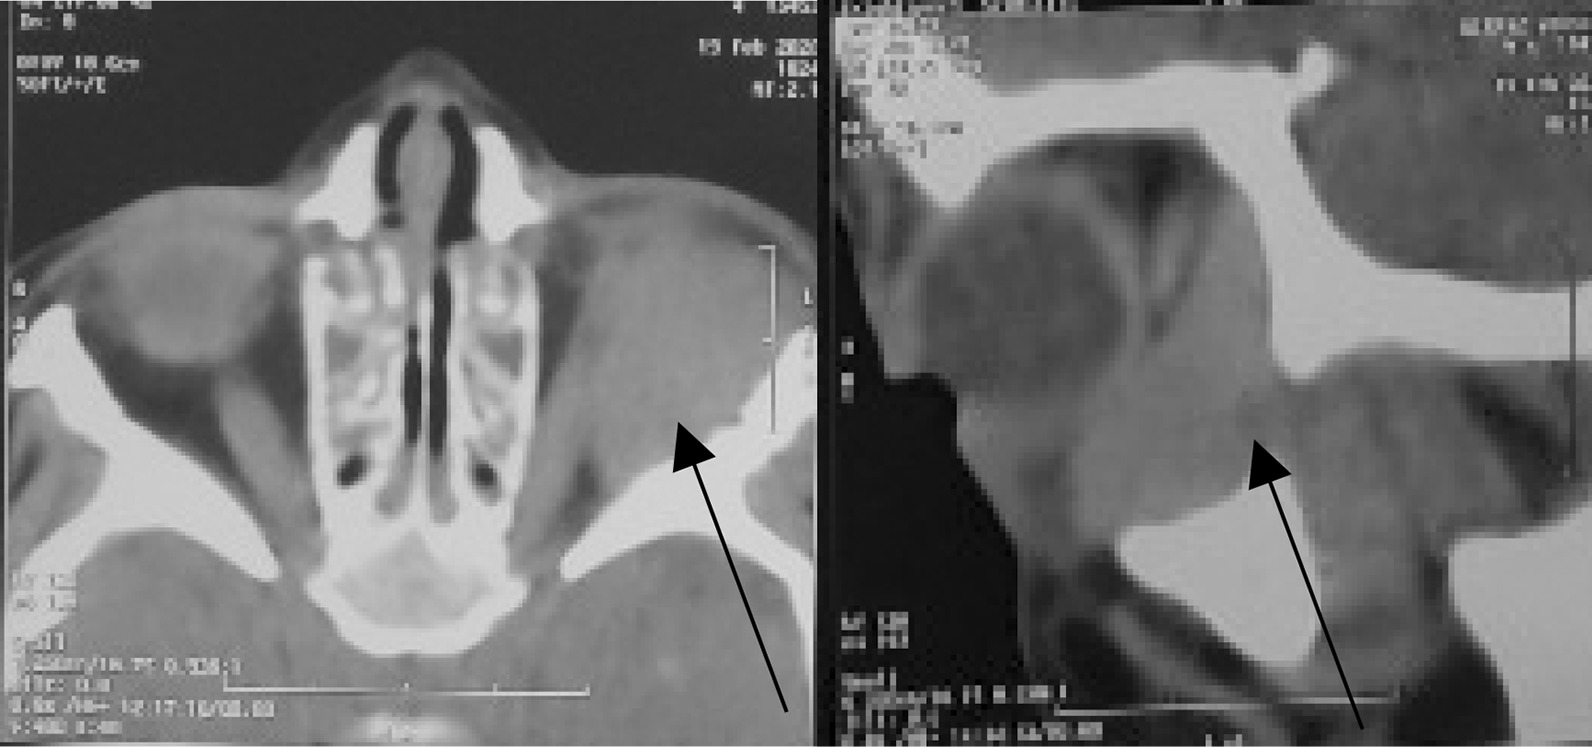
Craniofacial computed tomography showing a spontaneously hyperdense, oval-shaped, extraconal mass repressing the inferior rectus muscle of the left orbit (arrows)
